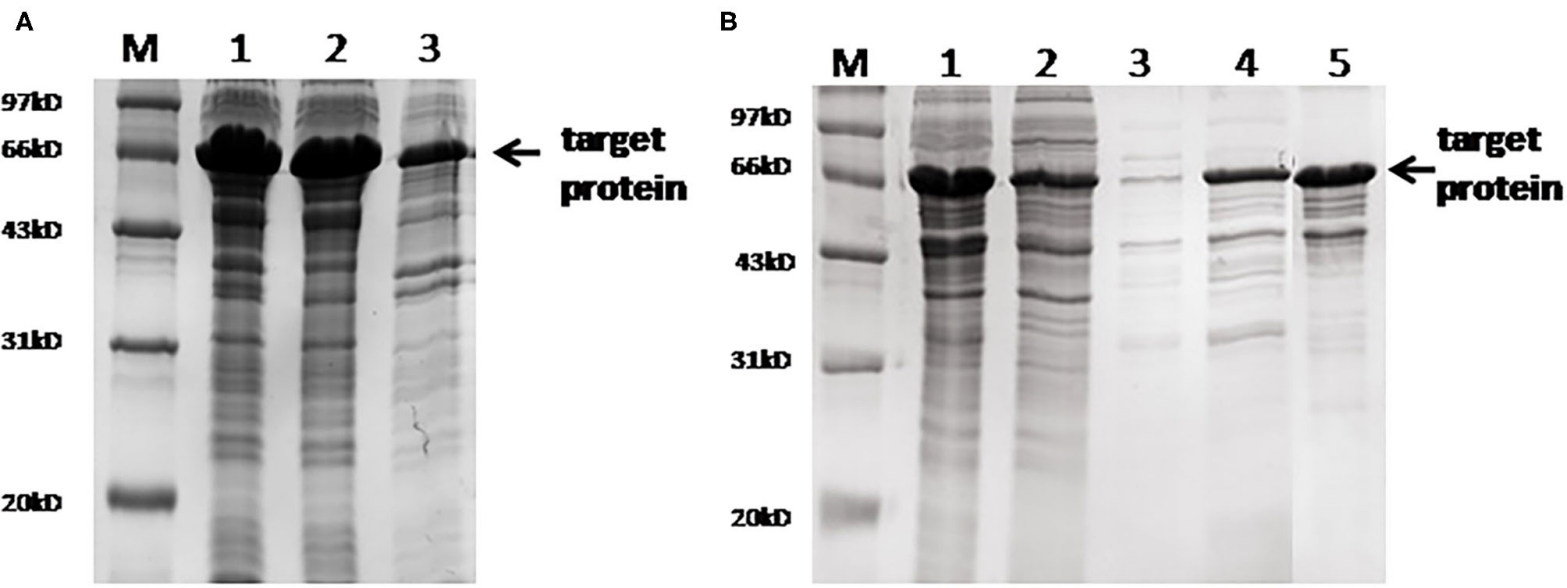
SDS-PAGE analysis of the fusion protein. (A) Protein expression results. M, marker; lane 1, whole bacteria after ultrasound; lane 2, supernatant after ultrasound; lane 3, precipitation after ultrasound. (B) SDS-PAGE after protein purification. M, marker; lane 1, the original protein before purification; lane 2, flow-through solution; lane 3, 15 mm of imidazole elution fraction; lane 4, 60 mm of imidazole elution fraction; lane 5, 300 mm of imidazole elution fraction.
